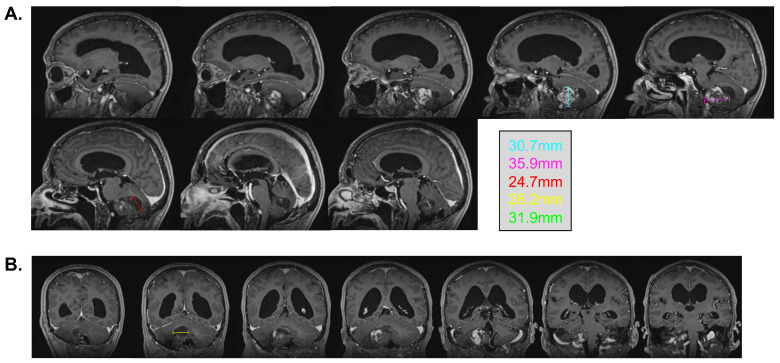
Pre-surgical MRI of the lesion. Representative capture of the T1-weighted MRI pre-surgical imaging. Representative measurements are color-coded. (A) Sagittal images are organized left to right and represent a left–right progression in the sagittal plane. (B) Coronal images are organized left to right and represent a posterior-to-anterior progression in the coronal plane.
